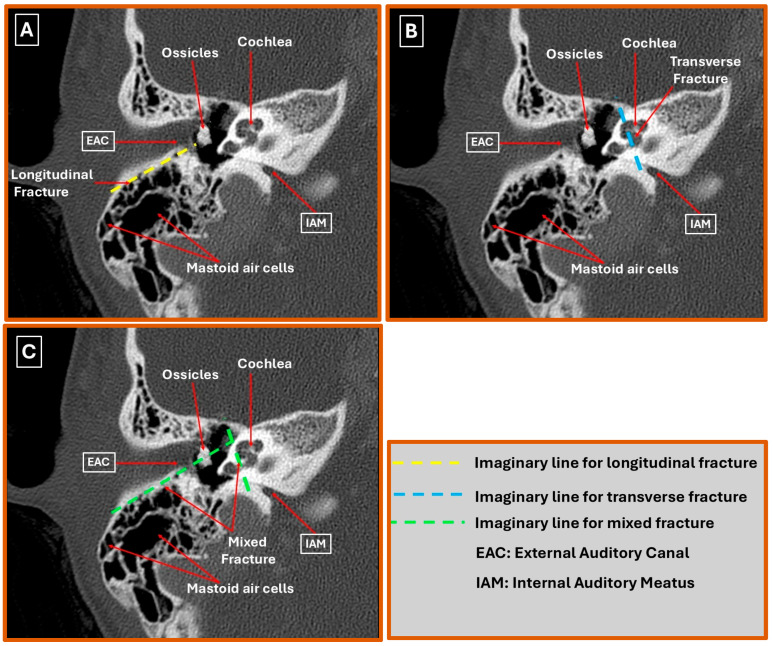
High-resolution CT patterns of temporal bone fractures in the right ear. (A) Longitudinal fracture: Lucent line parallels the petrous axis from the external acoustic meatus (EAM), sparing the otic capsule. (B) Transverse fracture: High-attenuation line traverses the petrous pyramid perpendicularly, breaching the internal acoustic meatus (IAM) and cochlear labyrinth (otic capsule-violating). (C) Mixed/oblique fracture: Intersecting fracture lines produce a stellate configuration that crosses both squamous and petrous cortices with associated ossicular dislocation and tympanic plate disruption.
